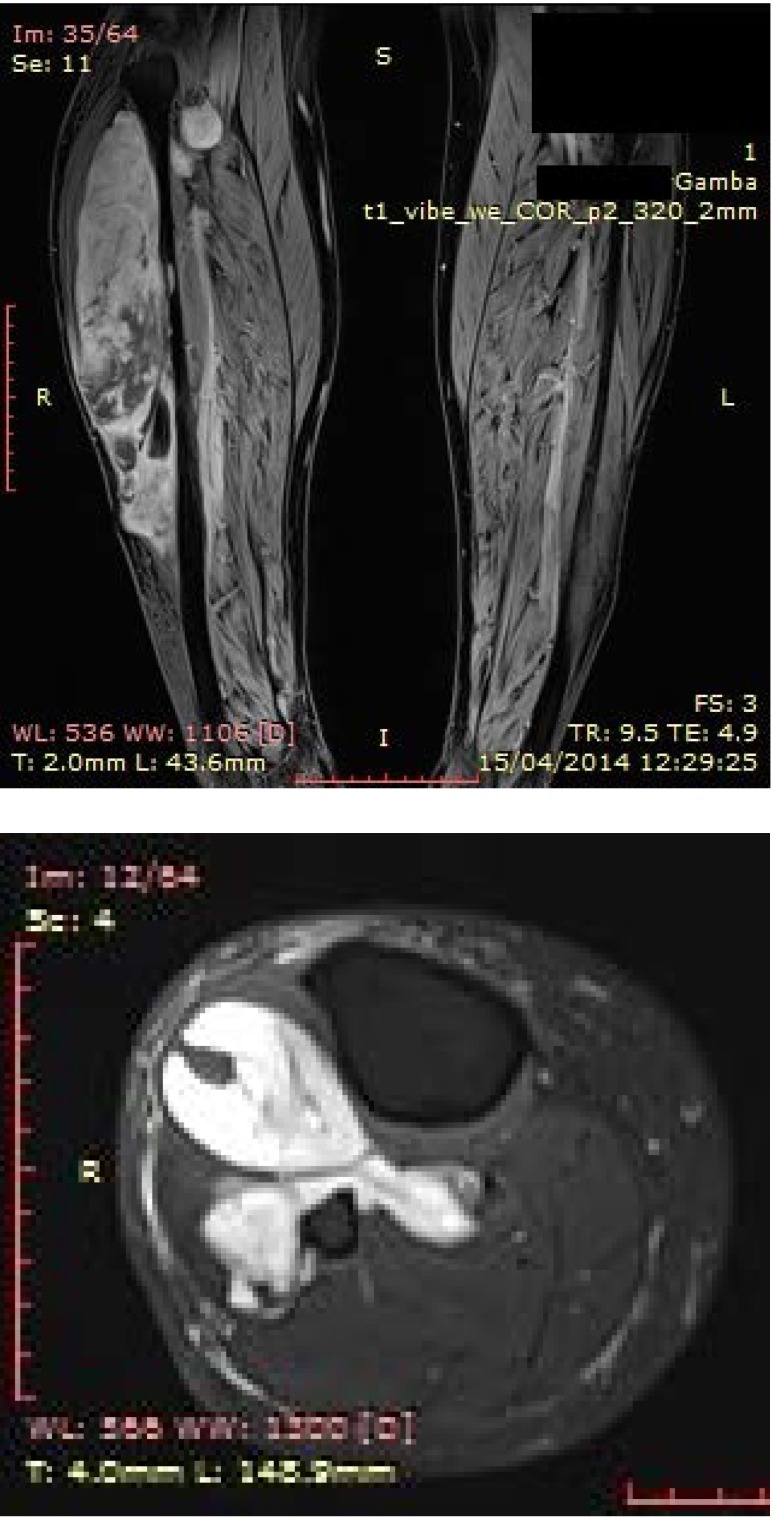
MRI- study: Soft tissue mass on the anterolateral aspect of the right calf, subsequent intratumoral necrosis, posterior compartment invasion (Personal photographic archive)
